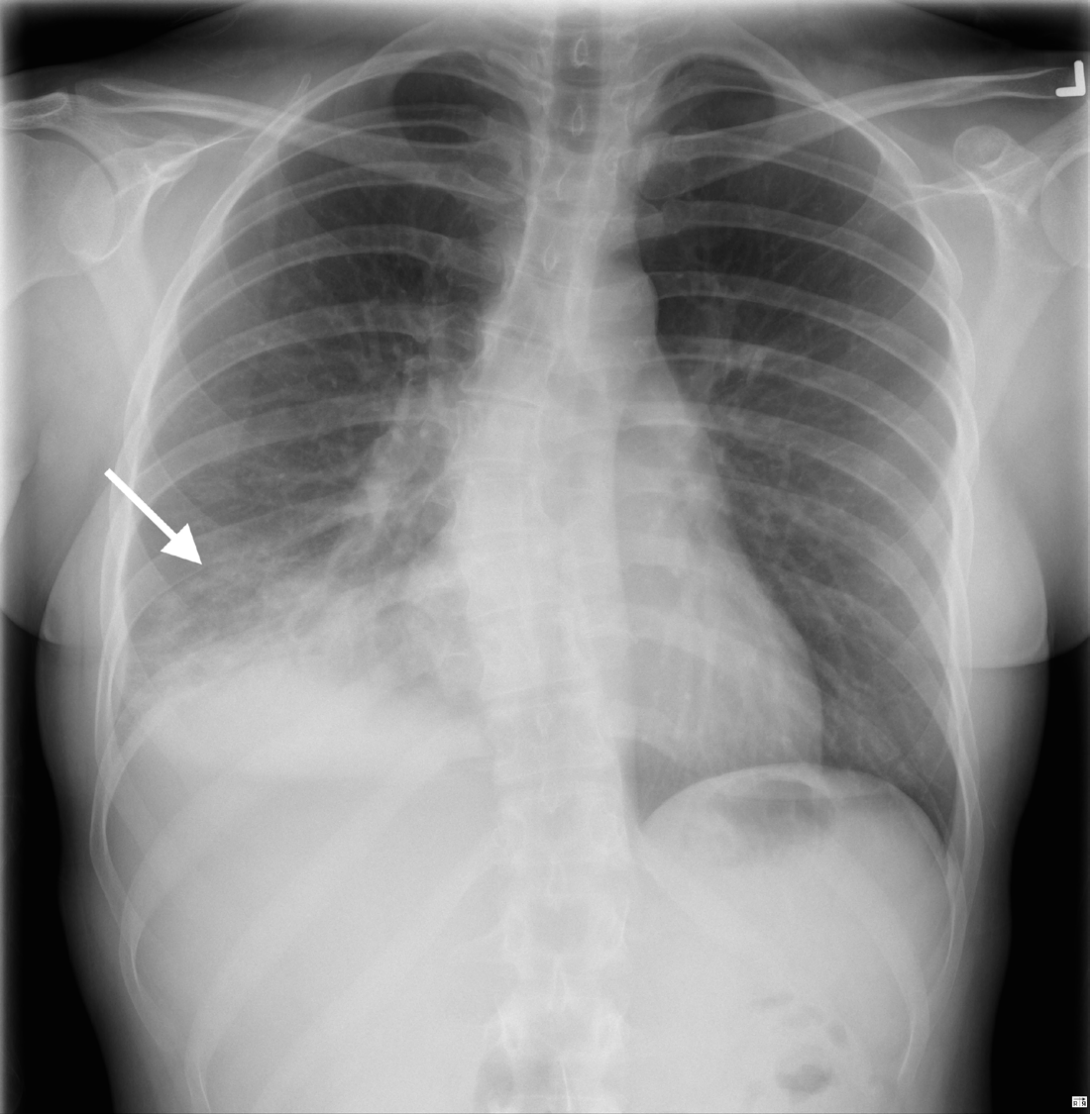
Anteroposterior portable chest radiograph: right lower lobe pneumonia.
